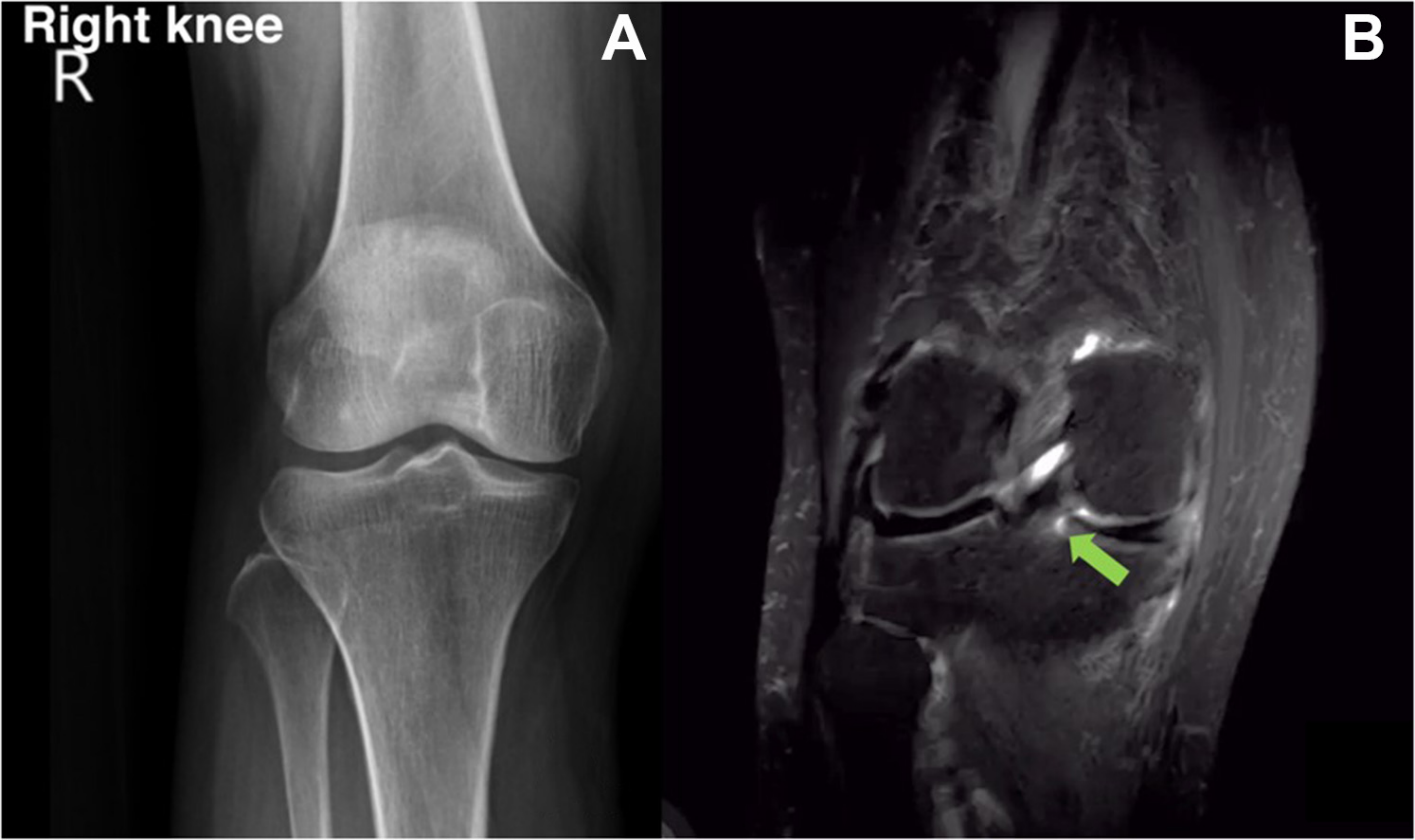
(A) One-leg standing anteroposterior view of the right (R) knee shows no medial joint space narrowing, malalignment of the knee, or osteoarthritis. (B) Magnetic resonance imaging (T2-weighted fat-suppressed coronal view) of the right knee shows that the medial meniscal root is completely torn, as represented by the cleft sign (green arrow).
